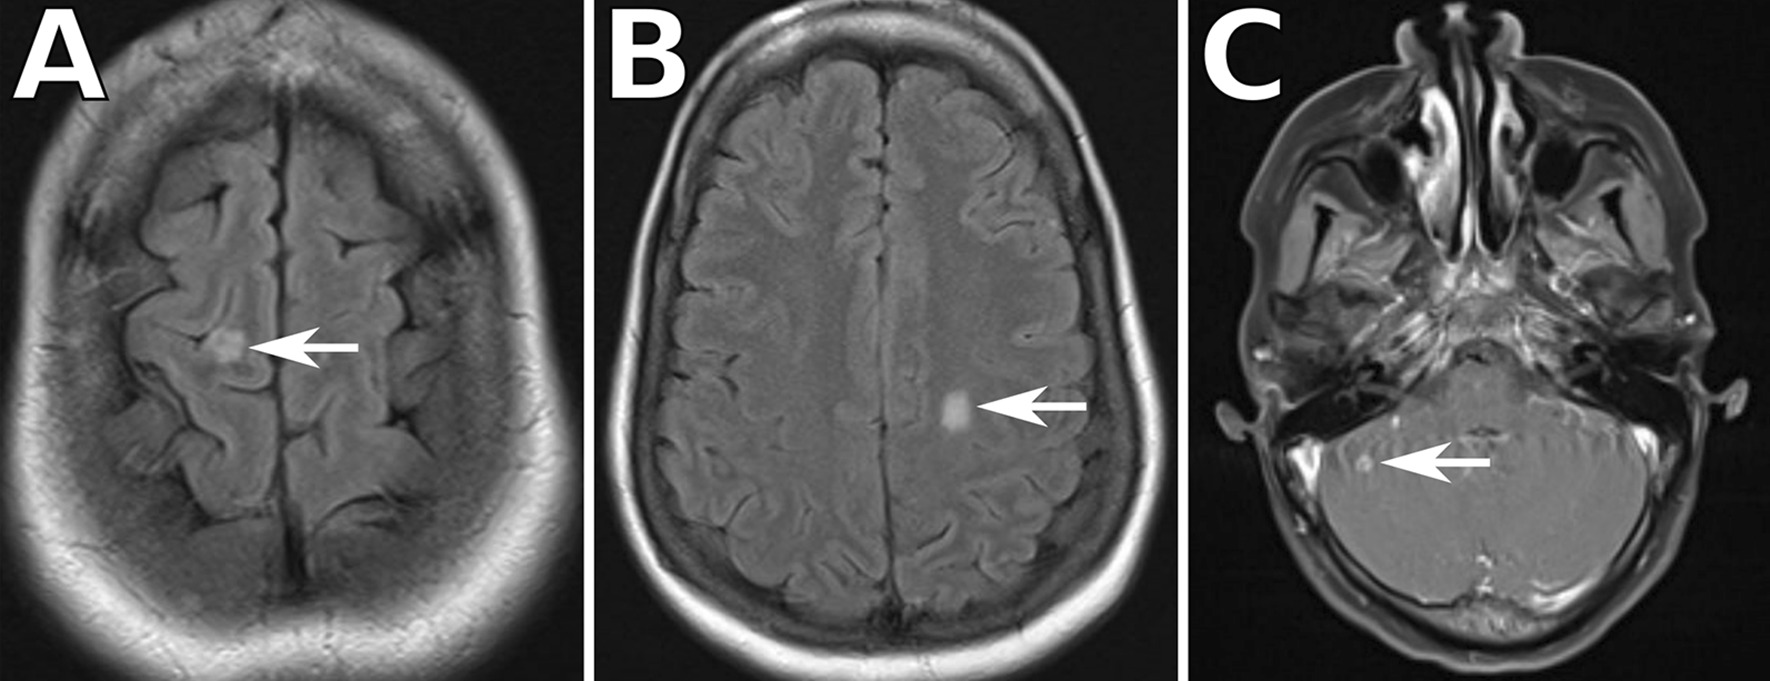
Magnetic resonance imaging of the brain showed A, B multiple supratentorial and C infratentorial enhancing lesions consistent with tuberculomas
